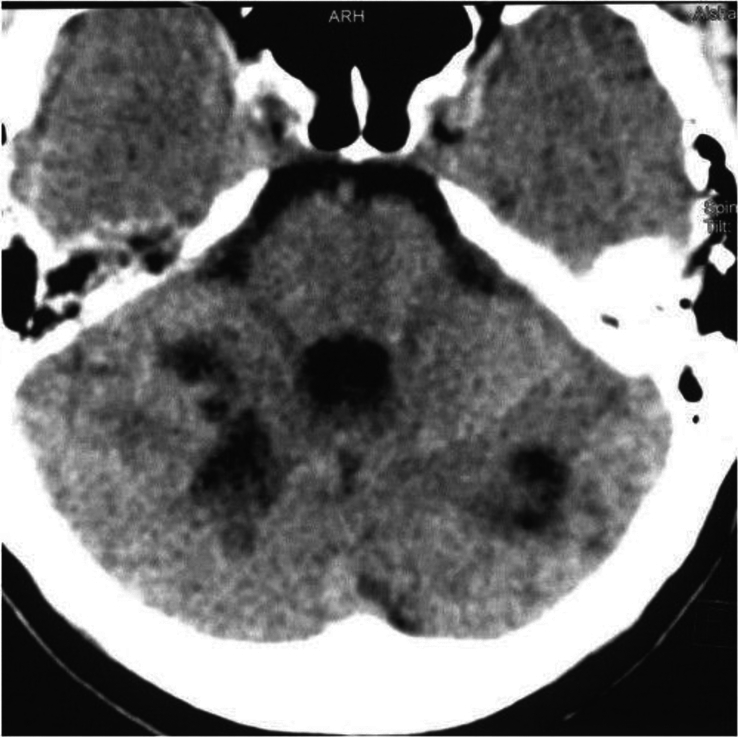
Brain computed tomography scan showing low-density areas located in both cerebellar hemispheres, indicative of cerebellar infarction.
